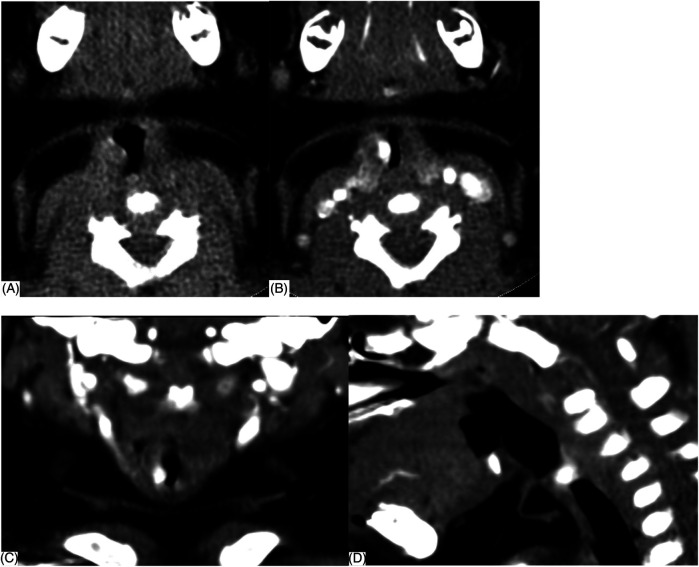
Contrast-enhanced CT (CECT) scan showing a well-defined enhancing lesion (arrow) in the submucosal location of the right lateral subglottic region. (A) Plain CT (B) transverse section, (C) coronal section, and (D) median sagittal section.
